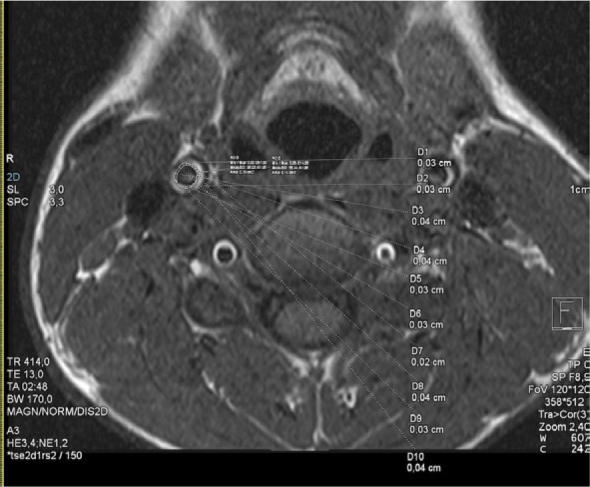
The MR imaging of the right common carotid artery. The measurements of carotid intima-media thickness (cIMT) in a healthy 14-year-old boy. The calculated average cIMT was 0.3 mm.
